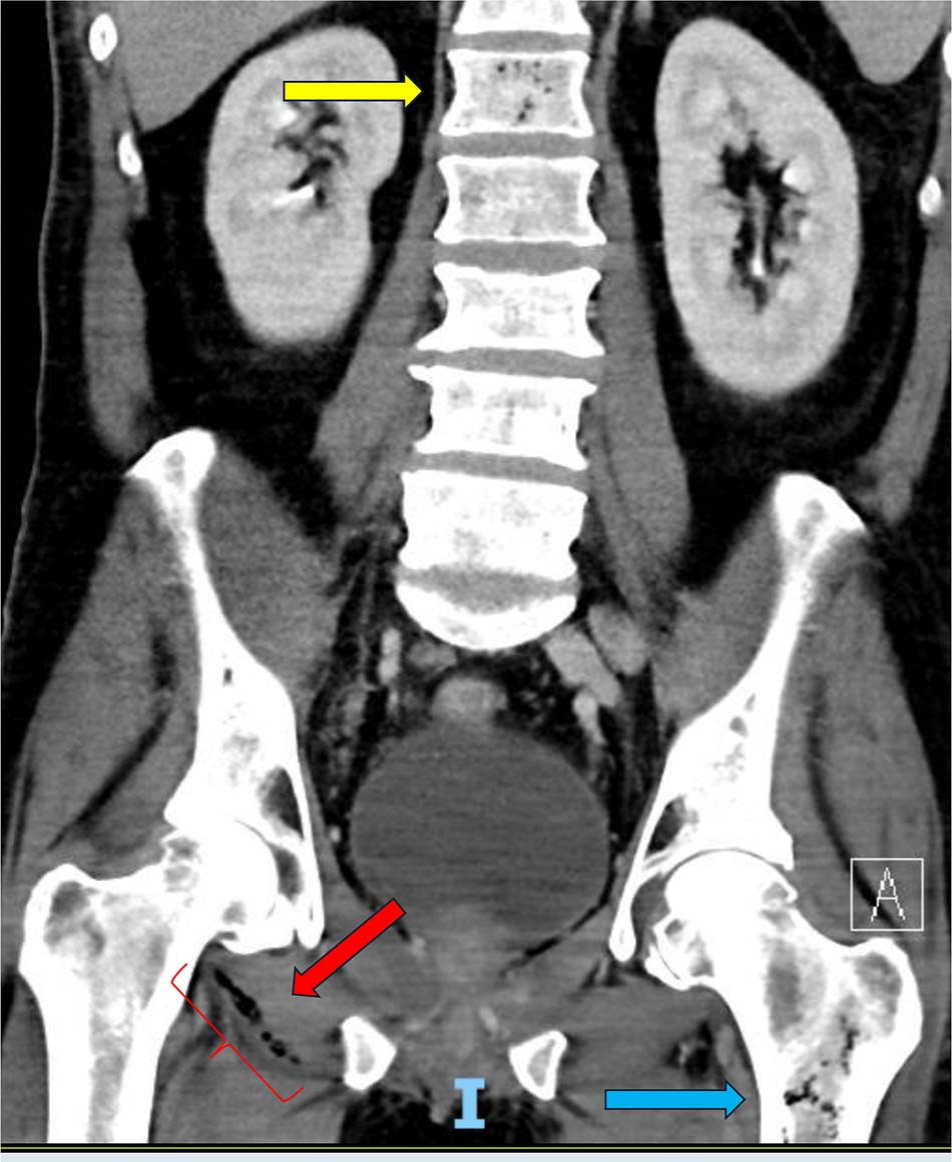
CT abdomen and pelvis—coronal plane. Intraosseous gas is visualized in the L1 vertebral body (yellow arrow, top middle) and left proximal femoral shaft to the level of the greater trochanter (blue arrow, bottom right). These sites are exemplary of the classic “pumice stone” sign, which is pathognomonic of emphysematous osteomyelitis [7]. Soft tissue gas is also seen in the right adductor longus (red arrow, bottom left). Soft tissue gas is commonly seen adjacent to involved bones in emphysematous osteomyelitis [7]. This patient has significant intraosseous gas of the right pubic ramus, although this is not visualized on this section.
